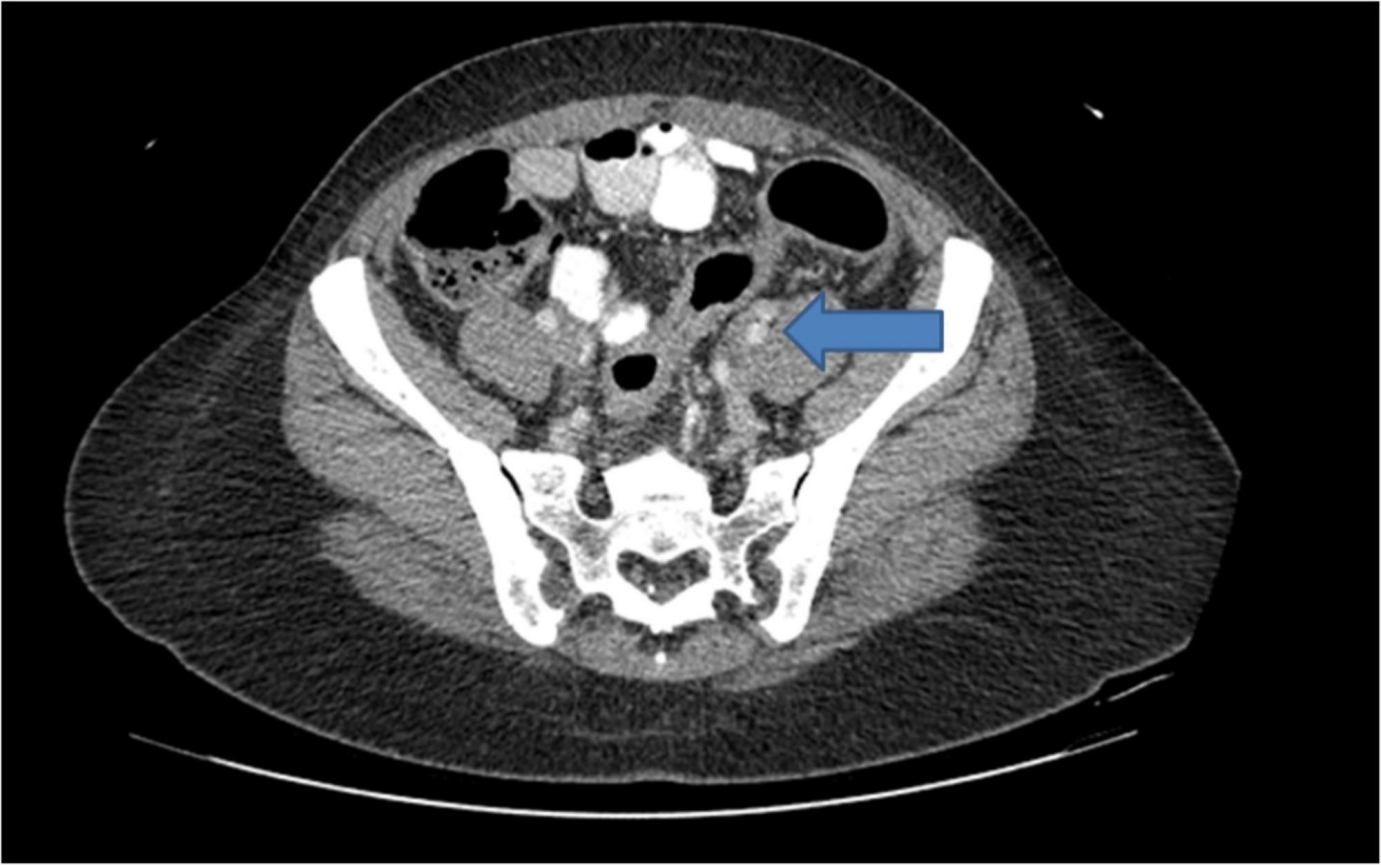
Computed Tomography of the Abdomen Circumferential wall thickening and mucosal hyperenhancement of the sigmoid colon and the rectum with surrounding inflammatory changes and several subcentimeter pericolonic lymph nodes (blue arrow).
